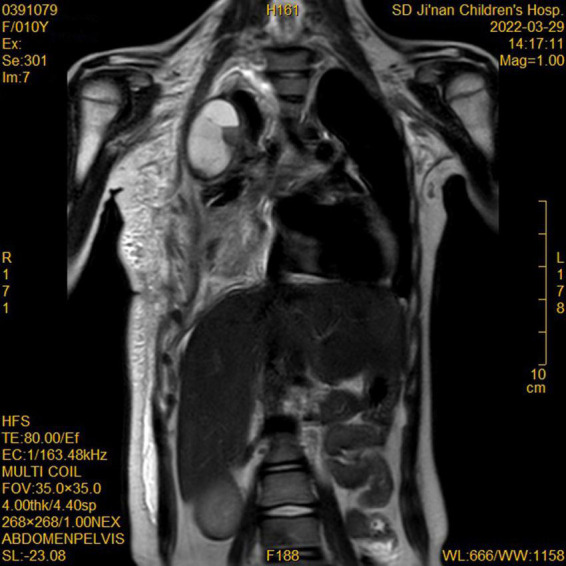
Magnetic resonance imaging (MRI) showed enhancement of chest wall lesions, atelectasis of the right lung, right encapsulated pleural effusion, and pleural thickening.
